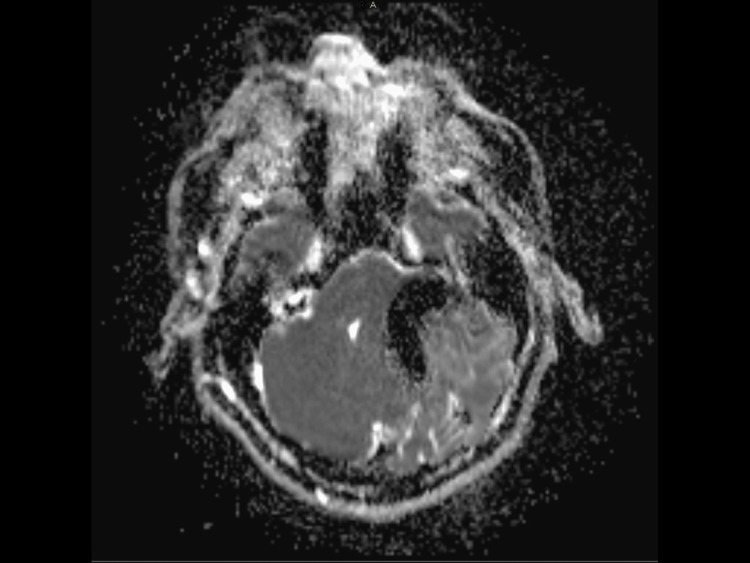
Preoperative MRI-ADC sequence showing diffusion restriction of the lesion content that was highly suggestive of an epidermoid cyst. MRI-ADC: Magnetic resonance imaging-Apparent diffusion coefficient
